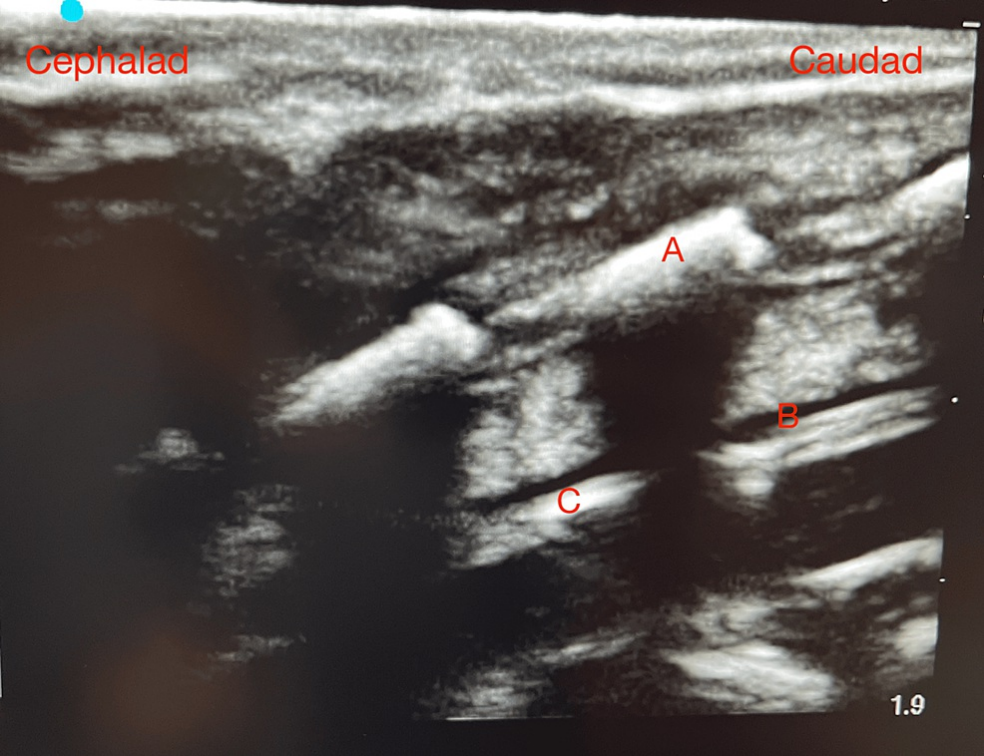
Ultrasound visualization of epidural needle placement. The different anatomic locations seen during ultrasound guidance insertion of the epidural catheter. The following landmarks were identified: (A) thoracic lamina, (B) epidural fluid, and (C) tip of the epidural catheter.
